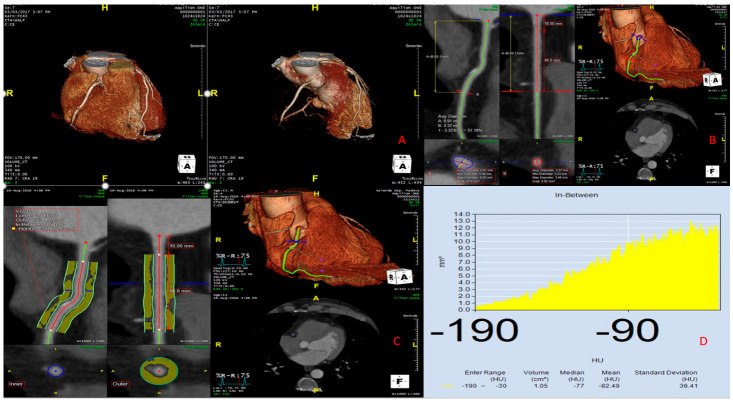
Example of how to measure the pericoronary adipose tissue. (A) Three-dimensional (3D) coronary CT reconstruction obtained using Aquarius TeraRecon. (B) Selection of the right coronary artery (RCA) and its region of interest using Aquarius TeraRecon. (C) Pericoronary tissue analysis showing the distribution of values between −190 and −30 HU. (D) Histogram showing the distribution of pericoronary tissue values in the range of −190 and −30 HU.
